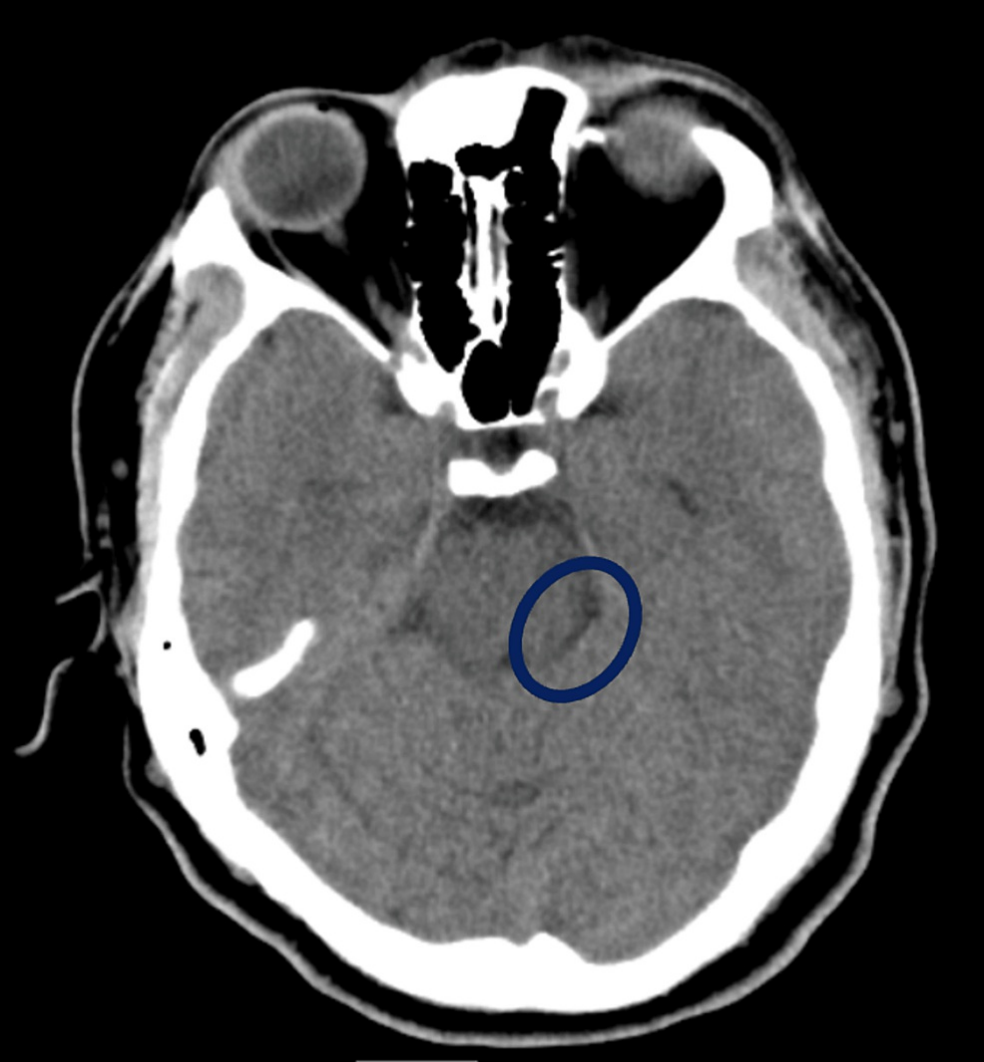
CT brain showing an ill-defined hypodensity in the left pontomedullary junction (blue circle)
